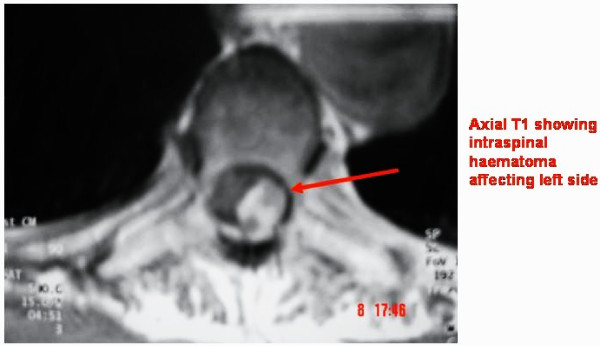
Pre-op MRI- T1 Axial showing intra-spinal haematoma at thoracic-5 level.
